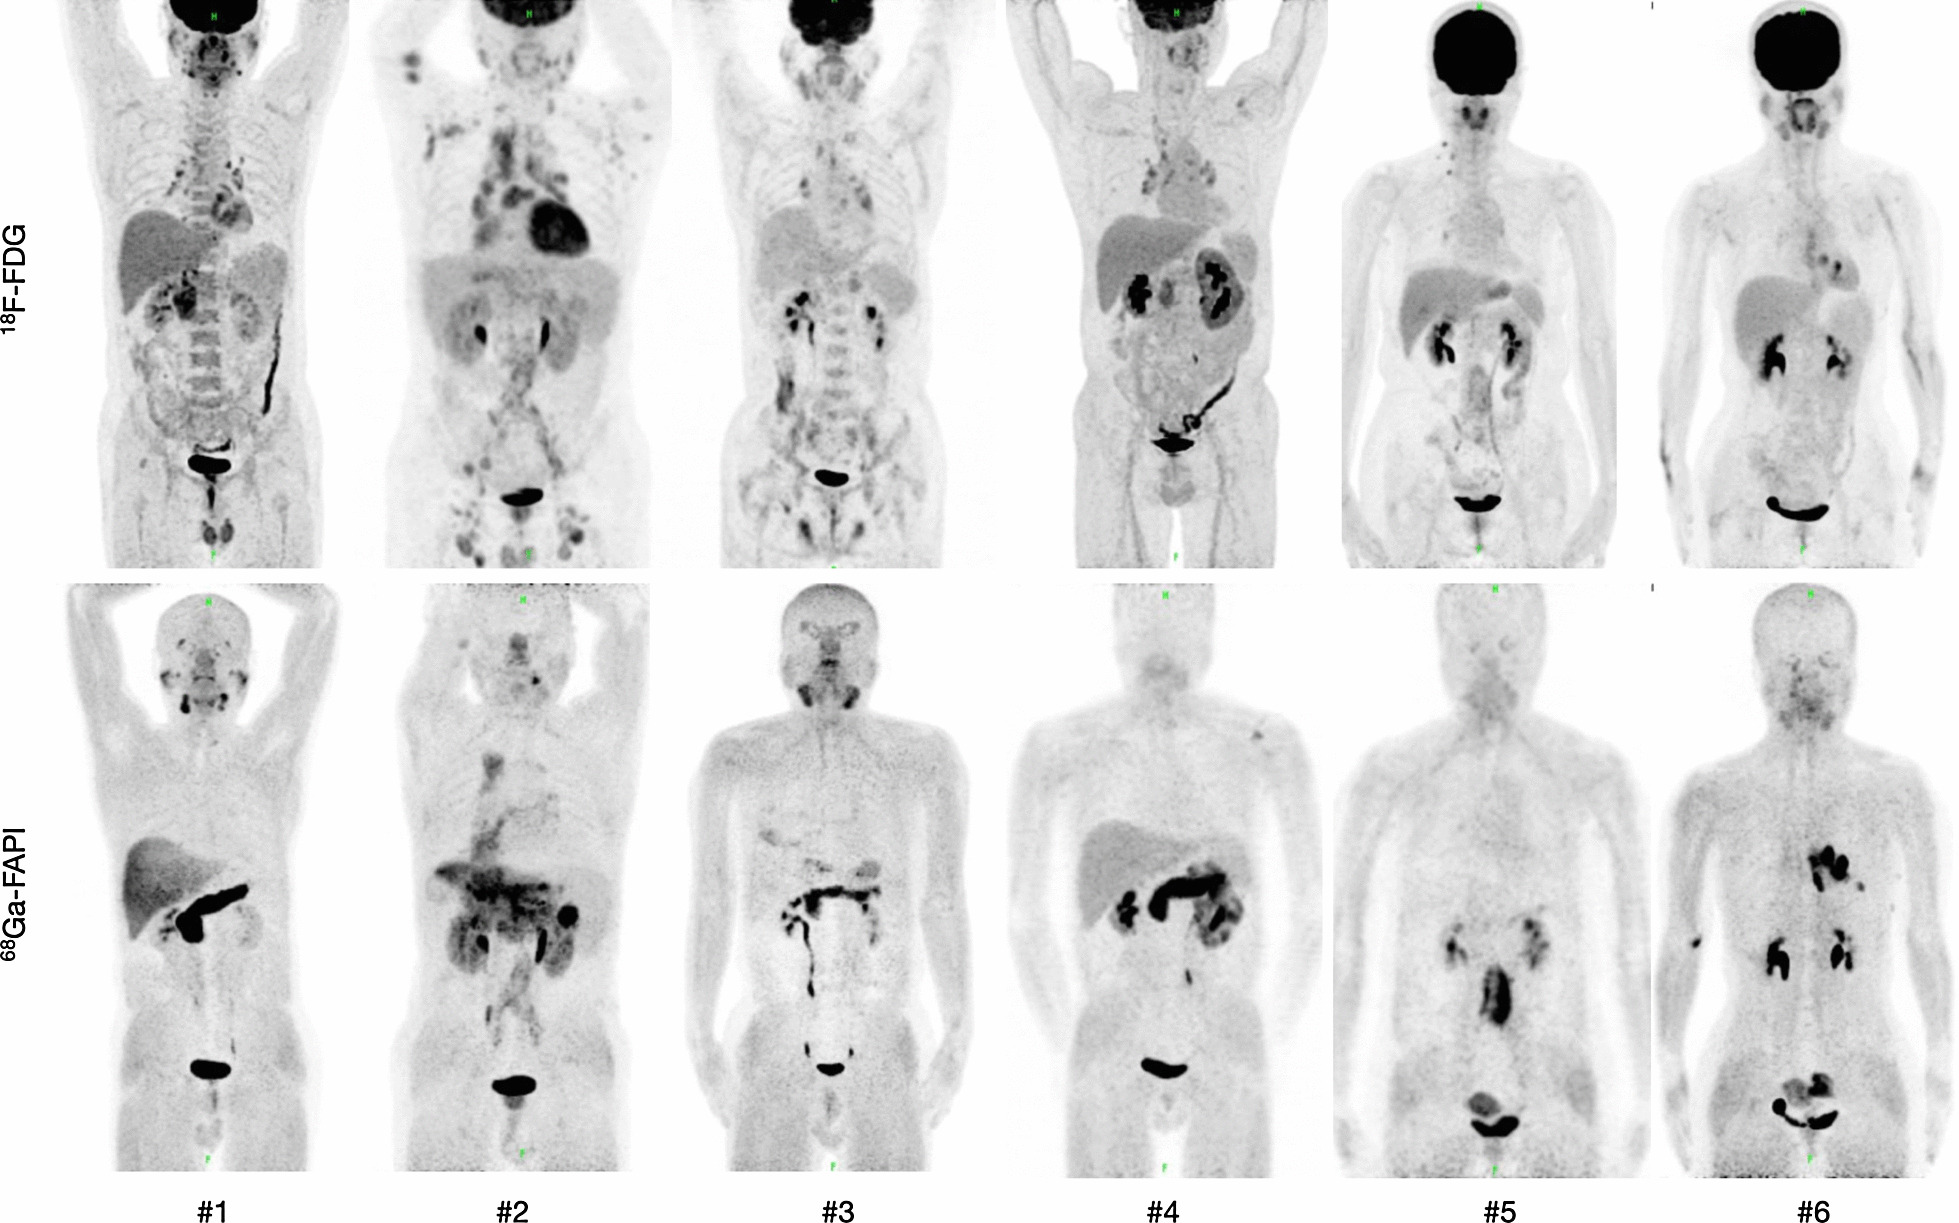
Comparison of 6 patients with IgG_4_-related disease (IgG_4_-RD) undergoing FDG and FAP-specific PET. FAP-specific PET detected IgG_4_-RD in the pancreas (patient #1, 2, 3, 4) bile duct/liver (patient #1, 2, 3), retroperitoneal fibrosis (patient #5), lung/pleura (patient #6), and salivary gland (patient #1, 3). Positive Iymph nodes on FDG PET show no enhancement on FAP-specific PET (patient #2, 3) (Fig. 2). This research was originally published in JNM. Luo et al. Fibroblast activation protein targeted PET/CT with ^68^Ga-FAPI for imaging IgG_4_-related disease: comparison to ^18^F-FDG PET/CT. J Nucl Med. 2020. ©SNMMI [47]
